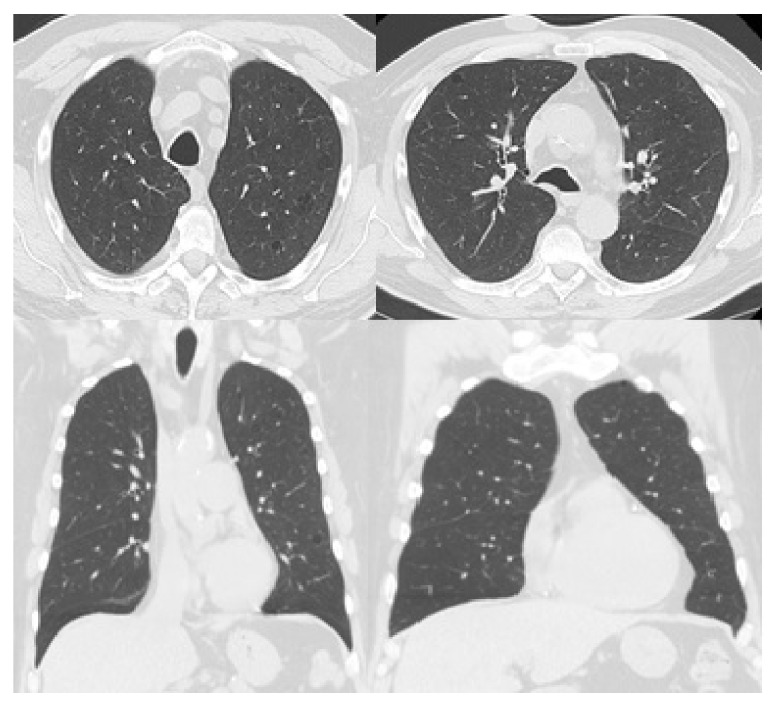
Follow-up HRCT images at the same level and planes as Figure 2 show resolution of the ground-glass nodules with persistent lung cysts.
